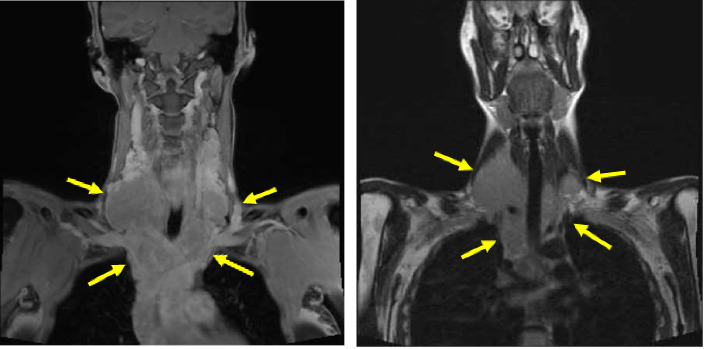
MRI of the neck shows bilateral (right greater than left) cervical and mediastinal lymphadenopathy (yellow arrows).
